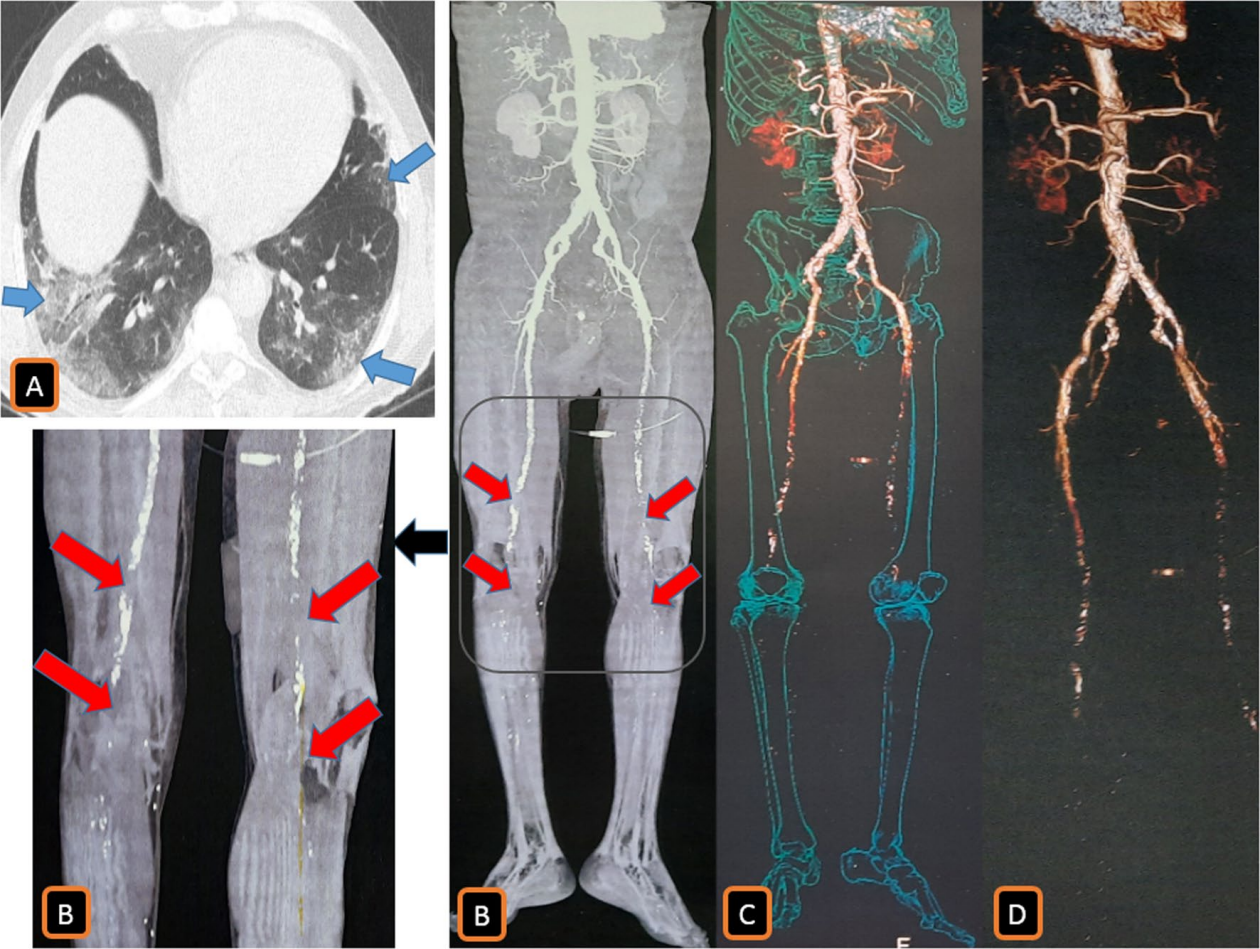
A 53-years-old male patient with a history of peripheral atherosclerosis. He presented to the emergency unit with acute severe bilateral leg pain and coldness. No chest symptoms were encountered. [A] Chest CT was urgently performed before admission, it revealed bilateral sub-pleural typical COVID-19 pneumonic ground-glass patches (blue arrows). CTA of the lower abdominal aorta and peripheral lower limbs was performed. [B–D] Surface shaded and colored VR images showed bilateral acute SFA and popliteal artery occlusion on top of heavy atherosclerotic changes with non-opacification of the popliteal trifurcation of infra-genicular arteries bilaterally (red arrows). PCR test result for COVID-19 infection was revealed to be positive and the D-dimer level was shooting (5125 ng/ml)
